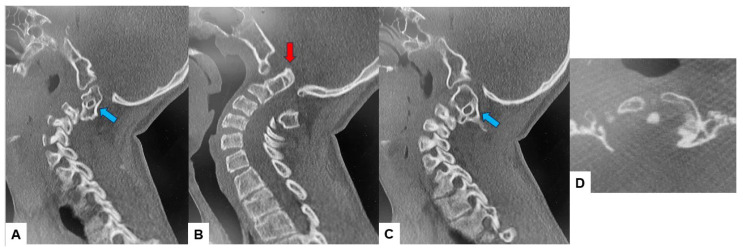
Preoperative CT, (A) Right sagittal reconstruction CT, (B) Mid-sagittal reconstruction CT, (C) Right sagittal reconstruction CT, (D) Axial CT at C1/2. The odontoid process was protruded into the foramen magnum (red arrow). C2 and C3 were fused (blue arrow).
